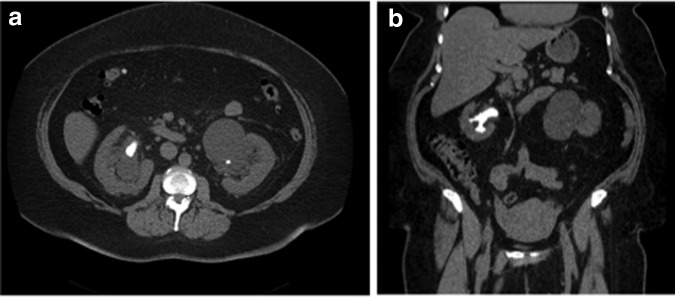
Preoperative axial and coronal stone protocol CT imaging. (a) Axial CT demonstrating presence of right staghorn calculus and left non-obstructing pelvic calculus. (b) Coronal CT demonstrating right staghorn calculus.
